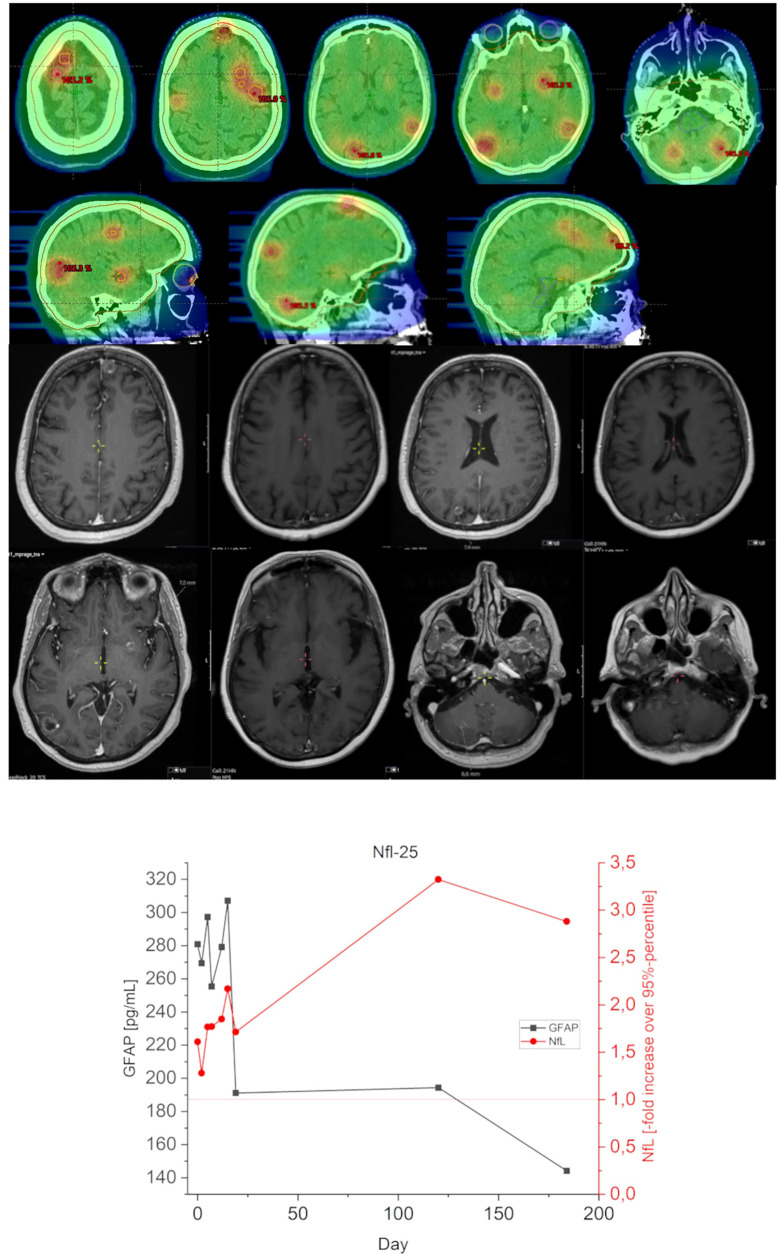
Patient NfL-25 treated with WBRT plus SiB to 15 brain metastases, with cranial MRI at first follow-up showing almost complete disappearance of all macroscopic lesions. NfL and GFAP serum values are displayed at the bottom of the figure.
